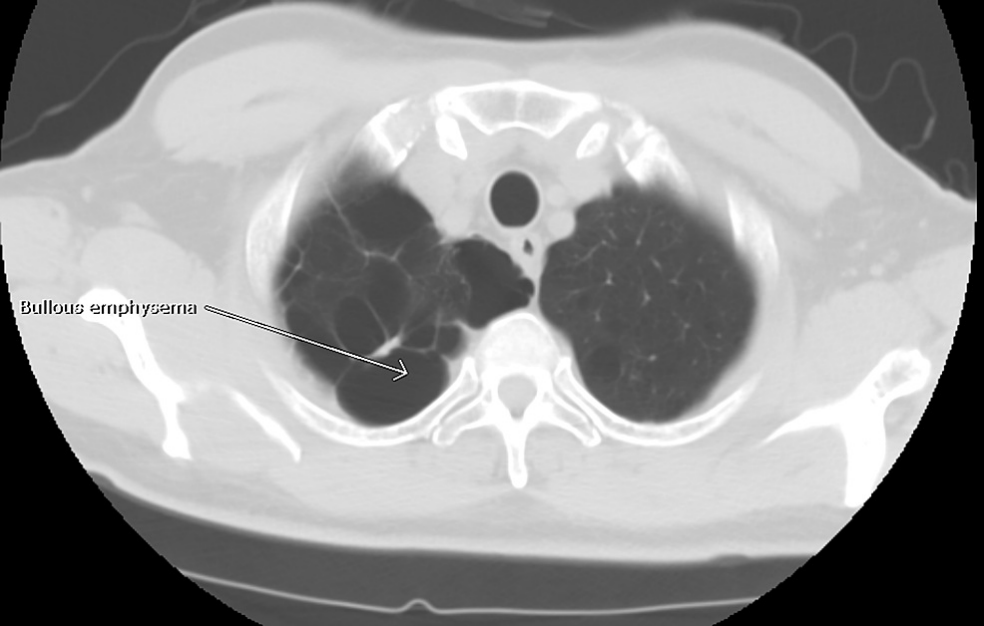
RUL apical bullous emphysema seen on chest CT scan
